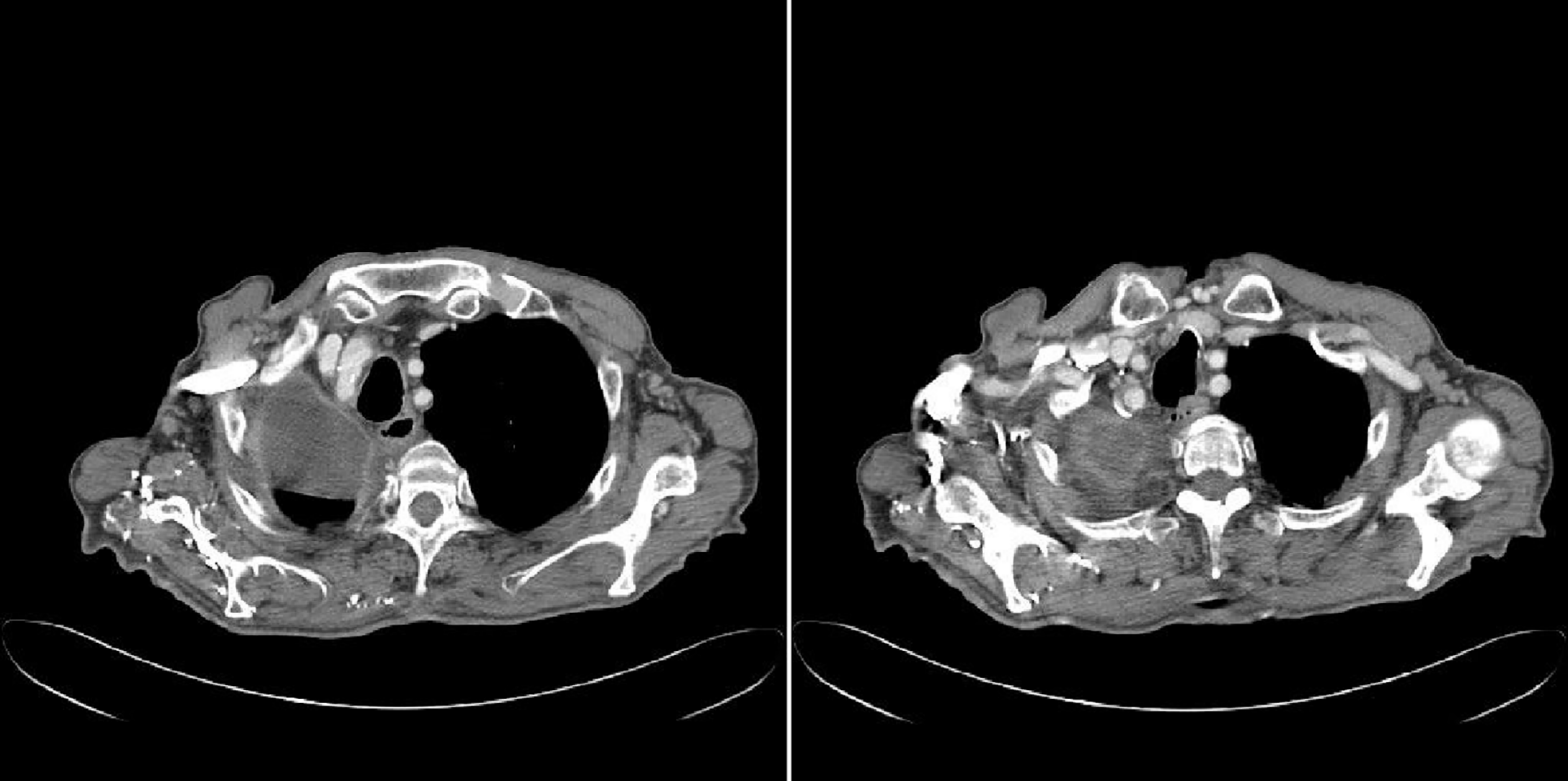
Postoperative total body computed tomography scan, negative for a residual mass.
